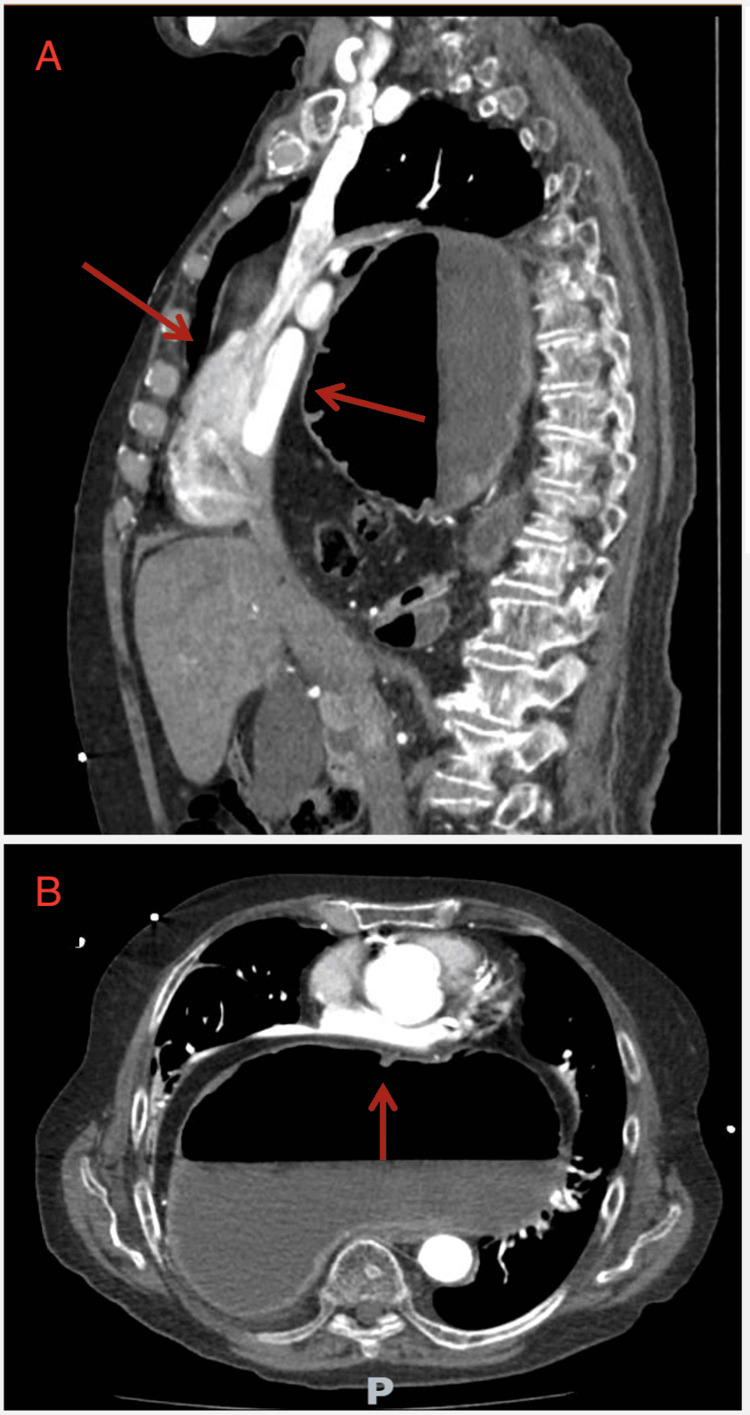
Sagittal (A) and Axial (B) view CT thorax. Pan-coronary atheromatous disease and compression of the heart (tamponade) due to massive overdistension of the hiatus hernia can be seen. Critical cardiac compromise was identified. Coronary artery patency was not evaluated on this CT study.
